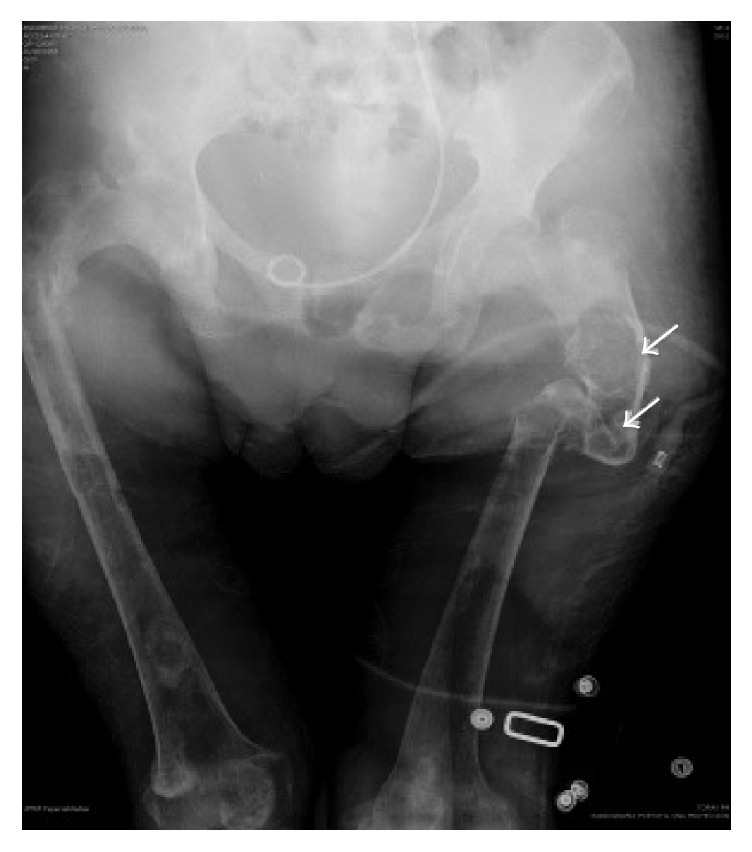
Pelvis X-ray with diffuse demineralization, marked decrease in cortical long bone, cysts, and brown tumors (arrows) and multiple pathological fractures for severe osteitis fibrous cystic.
